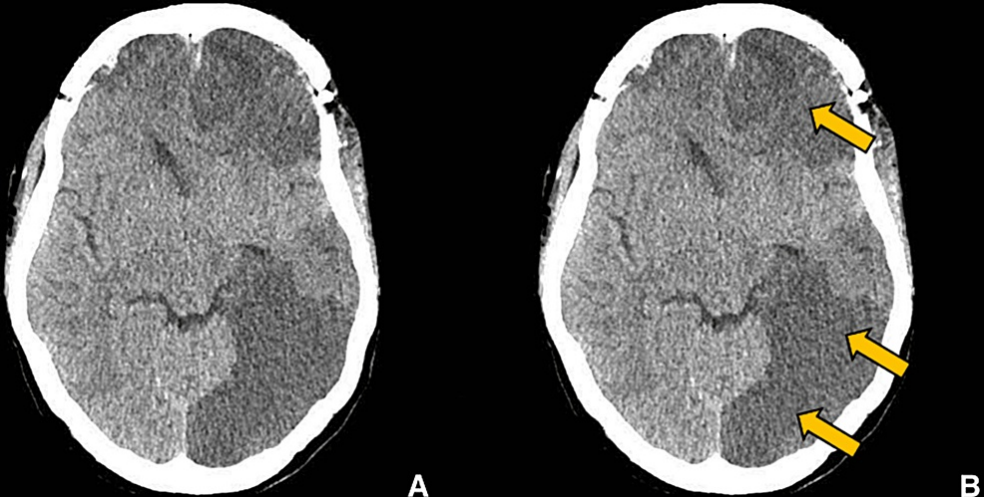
Postoperative imaging for patient 2. Non-contrast enhanced axial CT image showing multiple infarcts in bilateral middle cerebral artery and posterior cerebral artery territory. (A) Without ROI and (B) with ROI. The yellow arrows point to hypodense regions which show remnants of vessel infarction and decreased perfusion to the lesion following surgical debridement.
